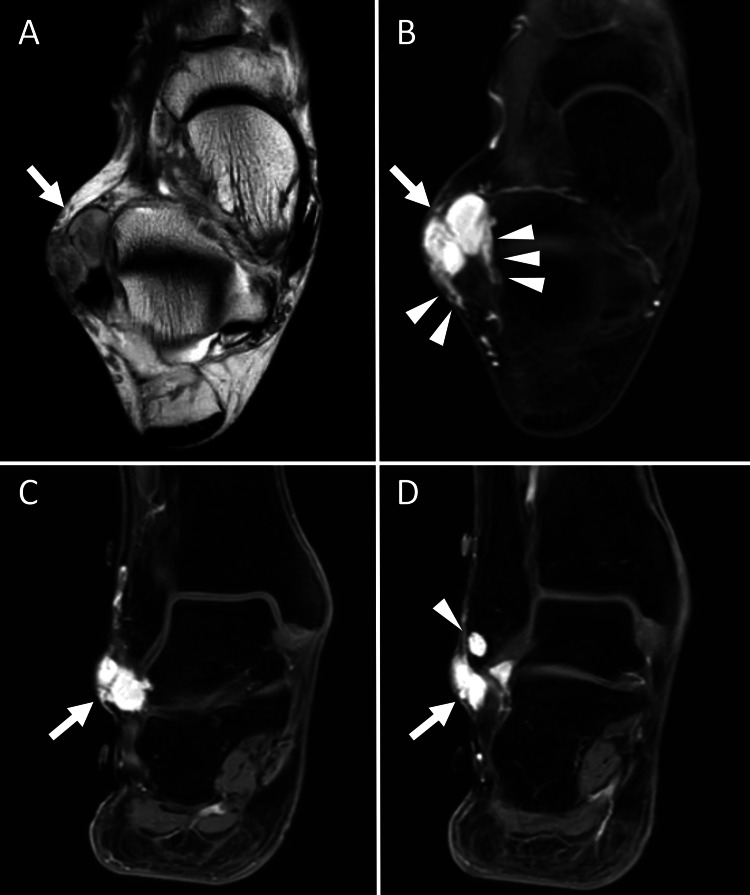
Magnetic resonance imaging findings. Axial T2-weighted image showed a well-defined, mildly hyperintense lesion in the subcutaneous tissue (A, arrow), and contrast-enhanced fat-suppressed T1-weighted MR image demonstrated marked and homogeneous enhancement of the lesion (B, arrow). Dilated blood vessels were observed around the lesion (B, arrowheads).  Coronal contrast-enhanced fat-suppressed T1-weighted MR images also revealed a prominently enhanced subcutaneous lesion over the right lateral malleolus (C, D, arrows), and a similar intraosseous lesion was found at the distal end of the fibula (D, arrowhead).
